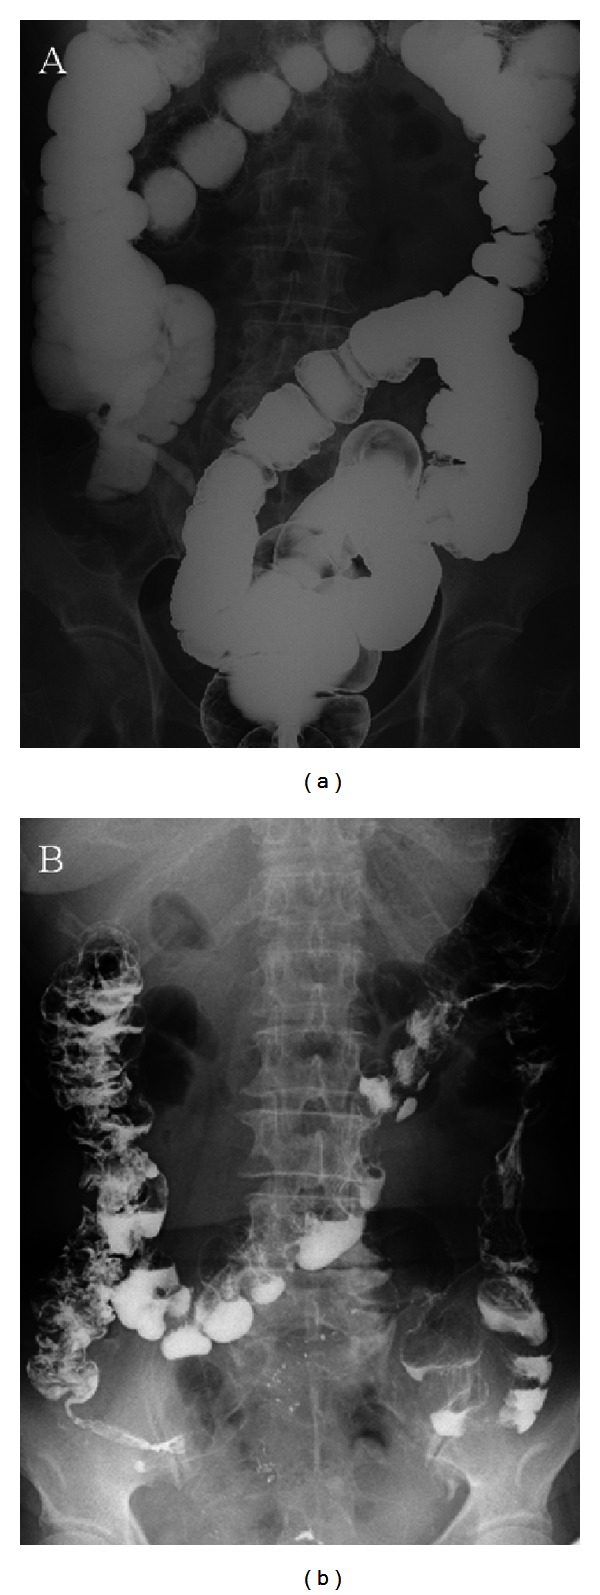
Barium radiography for colonic diverticular bleeding. (a) High-dose barium filling the entire colon by barium impaction therapy. (b) Abdominal radiograph showing barium retention in colonic diverticula taken the next day after barium impaction therapy.
